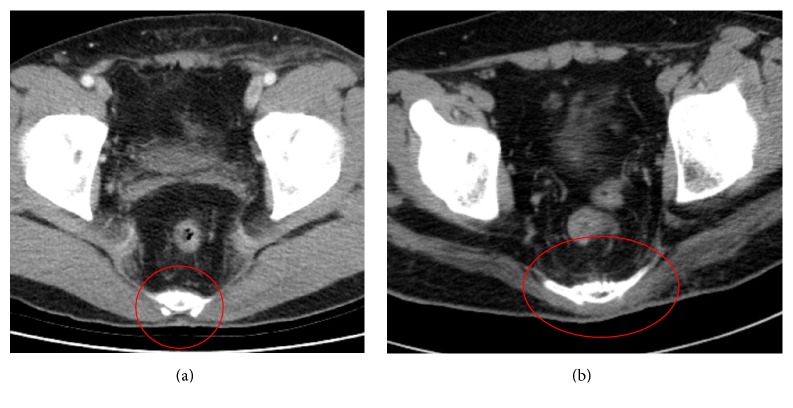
(a) Computed tomographic (CT) image of the sacrococcygeal joint of a healthy ambulatory individual. Gluteus muscle volume is well maintained, but the area around the sacrum appears thinned. (b) CT image of a patient who received modified gluteal fasciocutaneous flaps using a rotational muscle flap. The muscle appears well padded.
